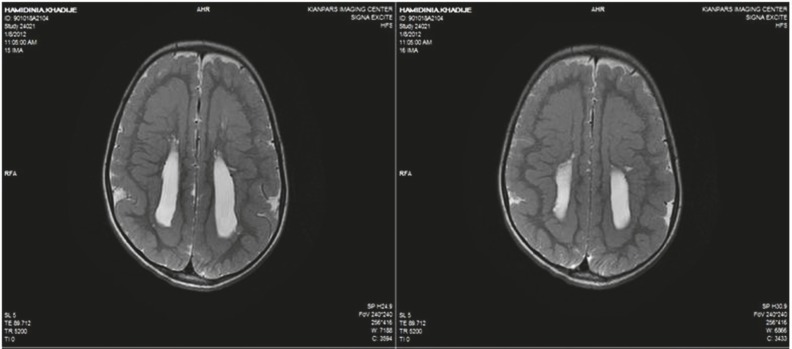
Axial T2-weighted brain MRI shows polymicrogyria, complete band heterotopia, and colpocephaly
